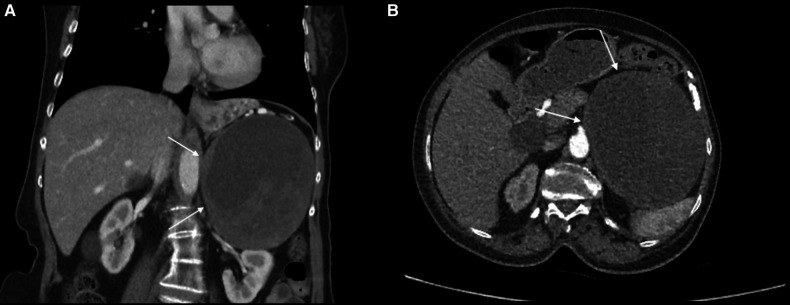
(A) left adrenal schwannoma in the coronal plane of the CT scan. The cystic necrotic component can be seen in this imaging study. The schwannoma was shown by a white arrow. (B) Left adrenal schwannoma in the axial plane of the CT scan. The cystic necrotic component can be seen in this imaging study. The schwannoma was shown by a white arrow.
